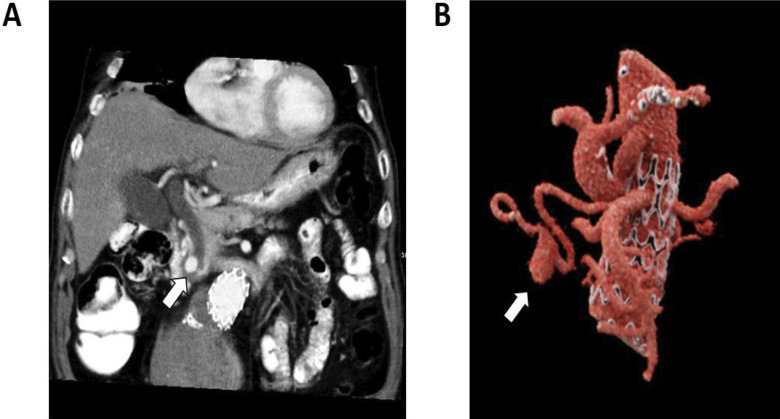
(A) Contrast-enhanced CT in an oblique coronal plan (MinIP recontruction) showing the right hepatic artery aneurysm at the level of the ampulla of Vater (arrow). Proximal dilation of the common bile duct is suggestive for obstruction. (B) VRT reconstruction showing a segment of the abdominal aorta with its branches: the celiac trunk, the superior mesenteric artery, from which the hepatic artery arises with the aneurysm (arrow), and the renal arteries.
